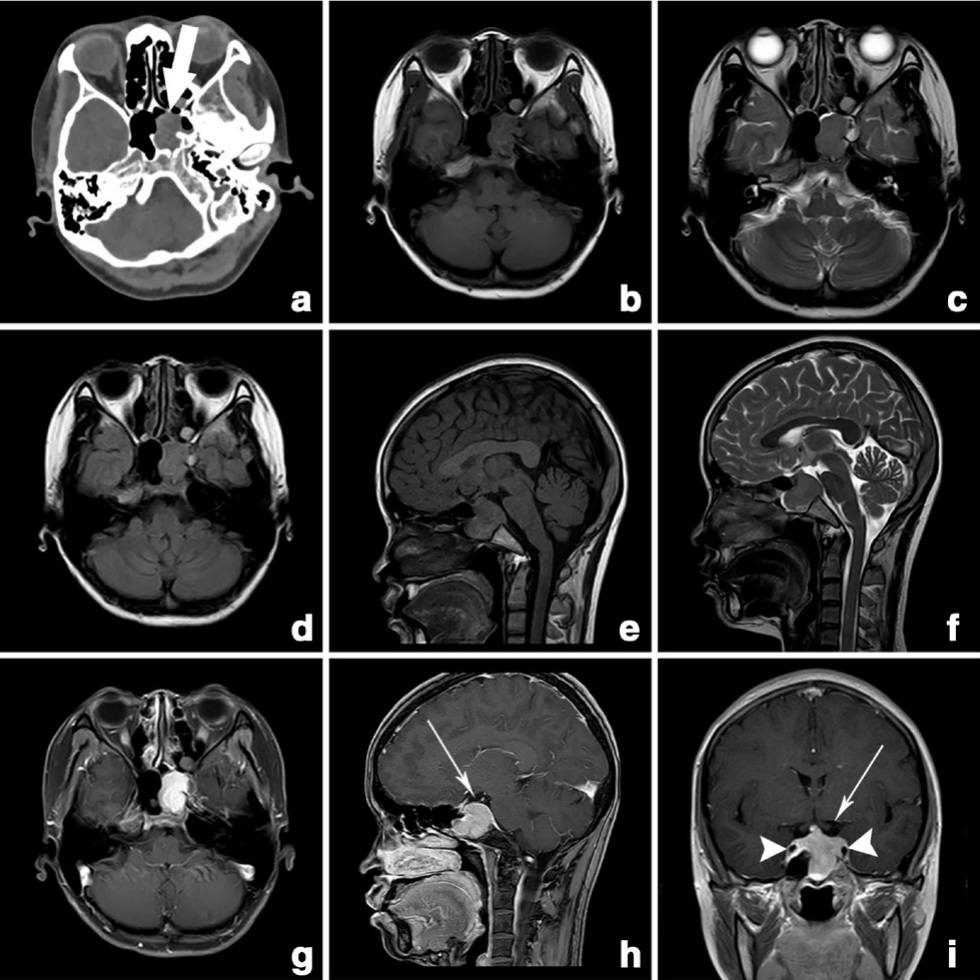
(a–i) Case 2: axial non-enhanced CT images (a) and MR images; axial *T*_1_WI (b), axial *T*_2_WI (c), axial FLAIR (d), sagittal *T*_1_WI (e), sagittal *T*_2_WI (f) and contrast enhanced *T*_1_WI axial (g), sagittal (h) and coronal (i) demonstrating expansile mass in the left sella turcica. The mass had low density with adjacent sphenoid bone destruction and cortical thinning (thick arrow) with displacement of optic chiasma (thin arrow) and bilateral internal carotid arteries (arrow heads). Contrast MR images demonstrated uniform enhancement in the lesion (g–i). FLAIR, fluid attenuated inversion recovery; *T*_1_WI, *T*_1_ weighted image; *T*_2_WI, *T*_2_weighted image.
